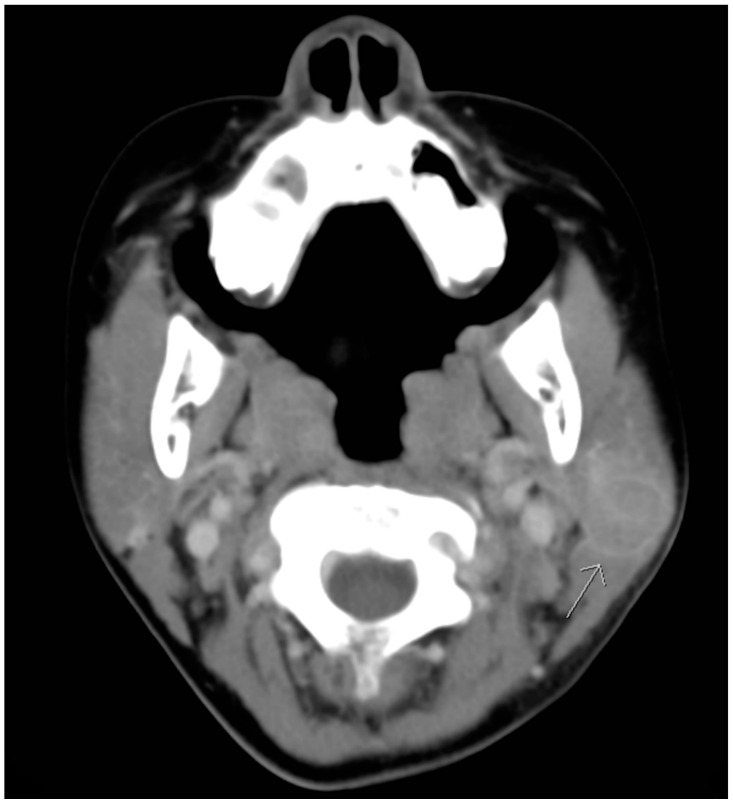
Transverse computed tomographic view revealing a hypodense cyst with ill-defined margin in the left parotid gland. (arrow head).
